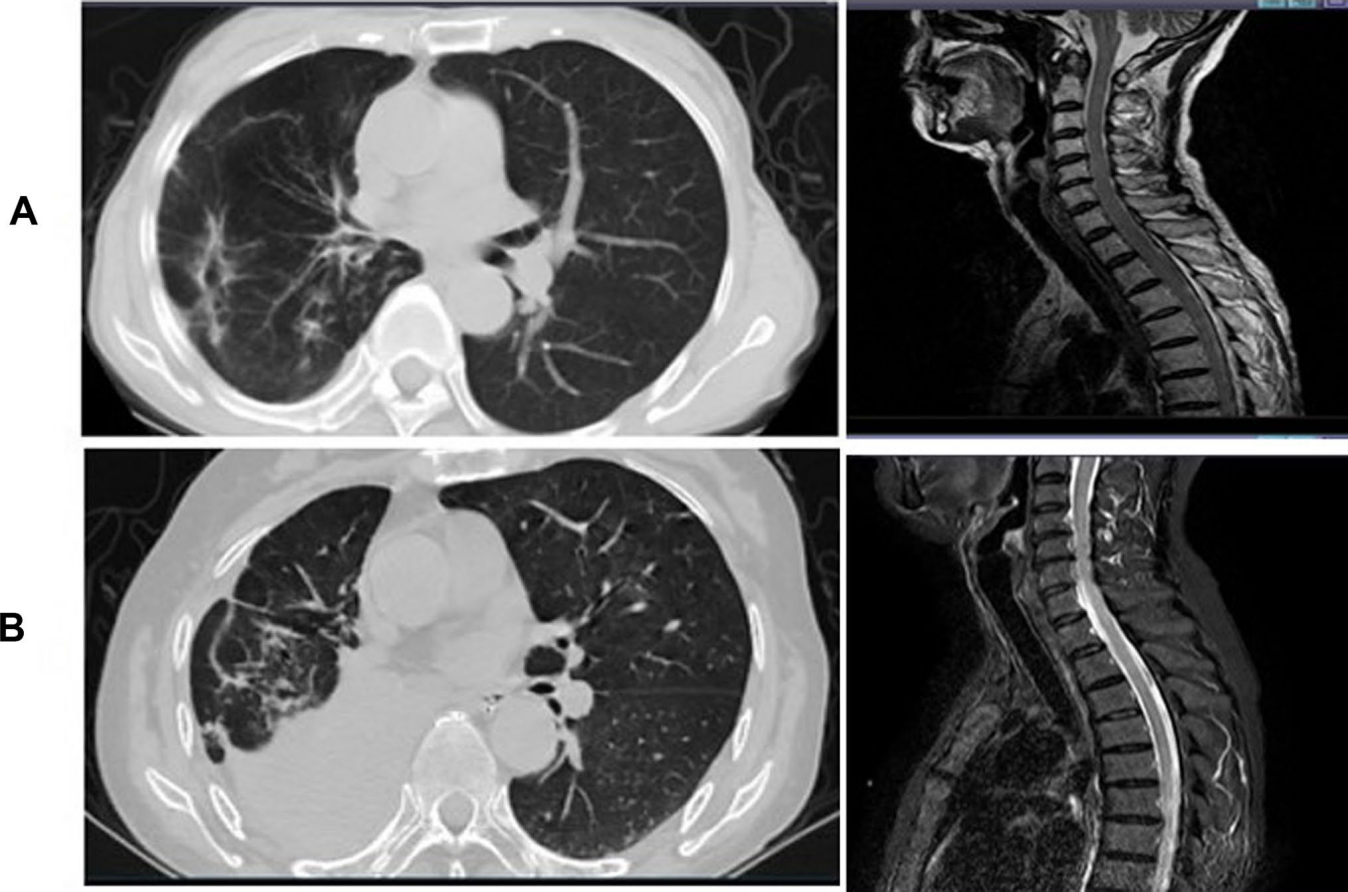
The progression of the lung tumor on chest CT were not synchronized with the changes of the cervicothoracic spinal cord on MRI. A After durvalumab treatment for three cycles. The right lower lobe lung cancer were obviously necrosis and formed thin-walled cavity formed, the hilar lymph nodes were significantly reduced. However, MRI of the spinal cord showed swellowed and ongitudinally extensive intramedullary hyperintensity in the cervicothoracic spinal cord. B The cancer progressed after cessation of anti-cancer therapy four months later. However, MRI showed atrophy of myelopathy.lesions of cervicothoracic spinal cord were stable
